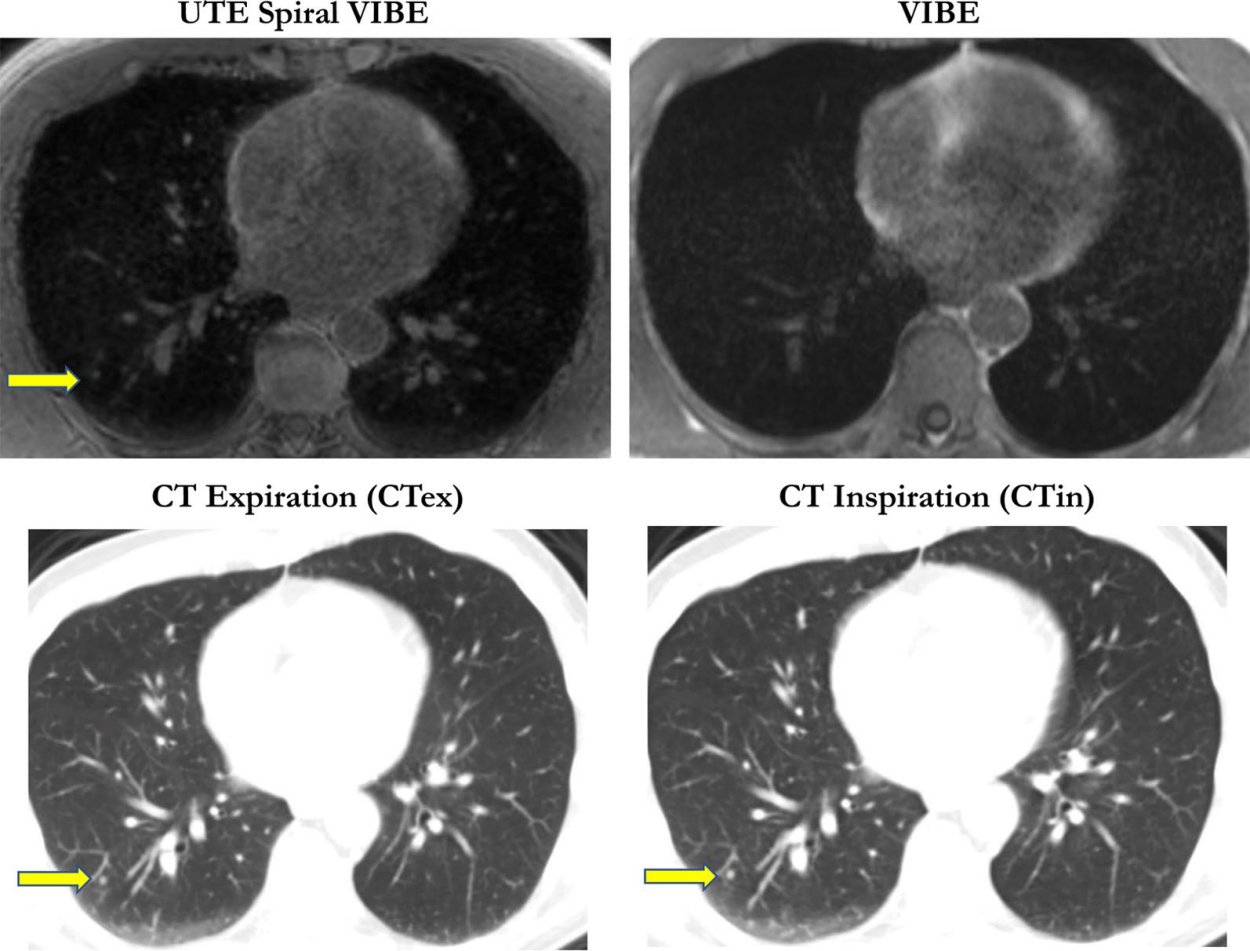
Pulmonary nodule in the right lower lobe was detected in UTE Spiral VIBE and both CT examinations (arrow; 3 mm). However, the lesion was not visible in VIBE in a patient with rectal carcinoma
